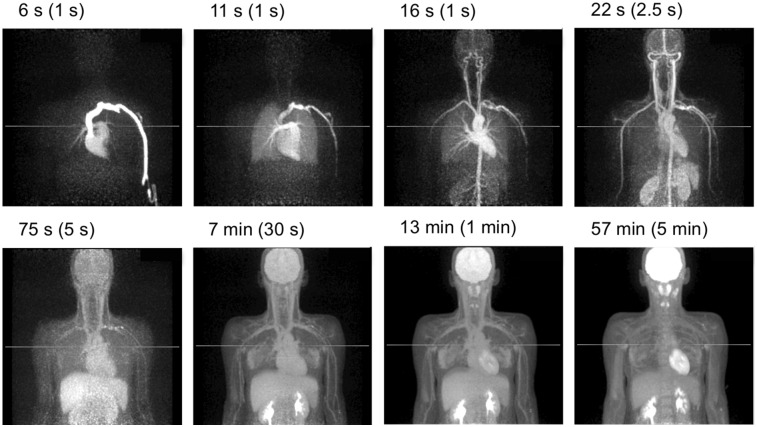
Dynamic ^18^F-FDG study of subject 7 acquired for 60 min after 555-MBq dose of ^18^F-FDG. Representative maximum-intensity projections are shown from 0 to 60 min after injection.
